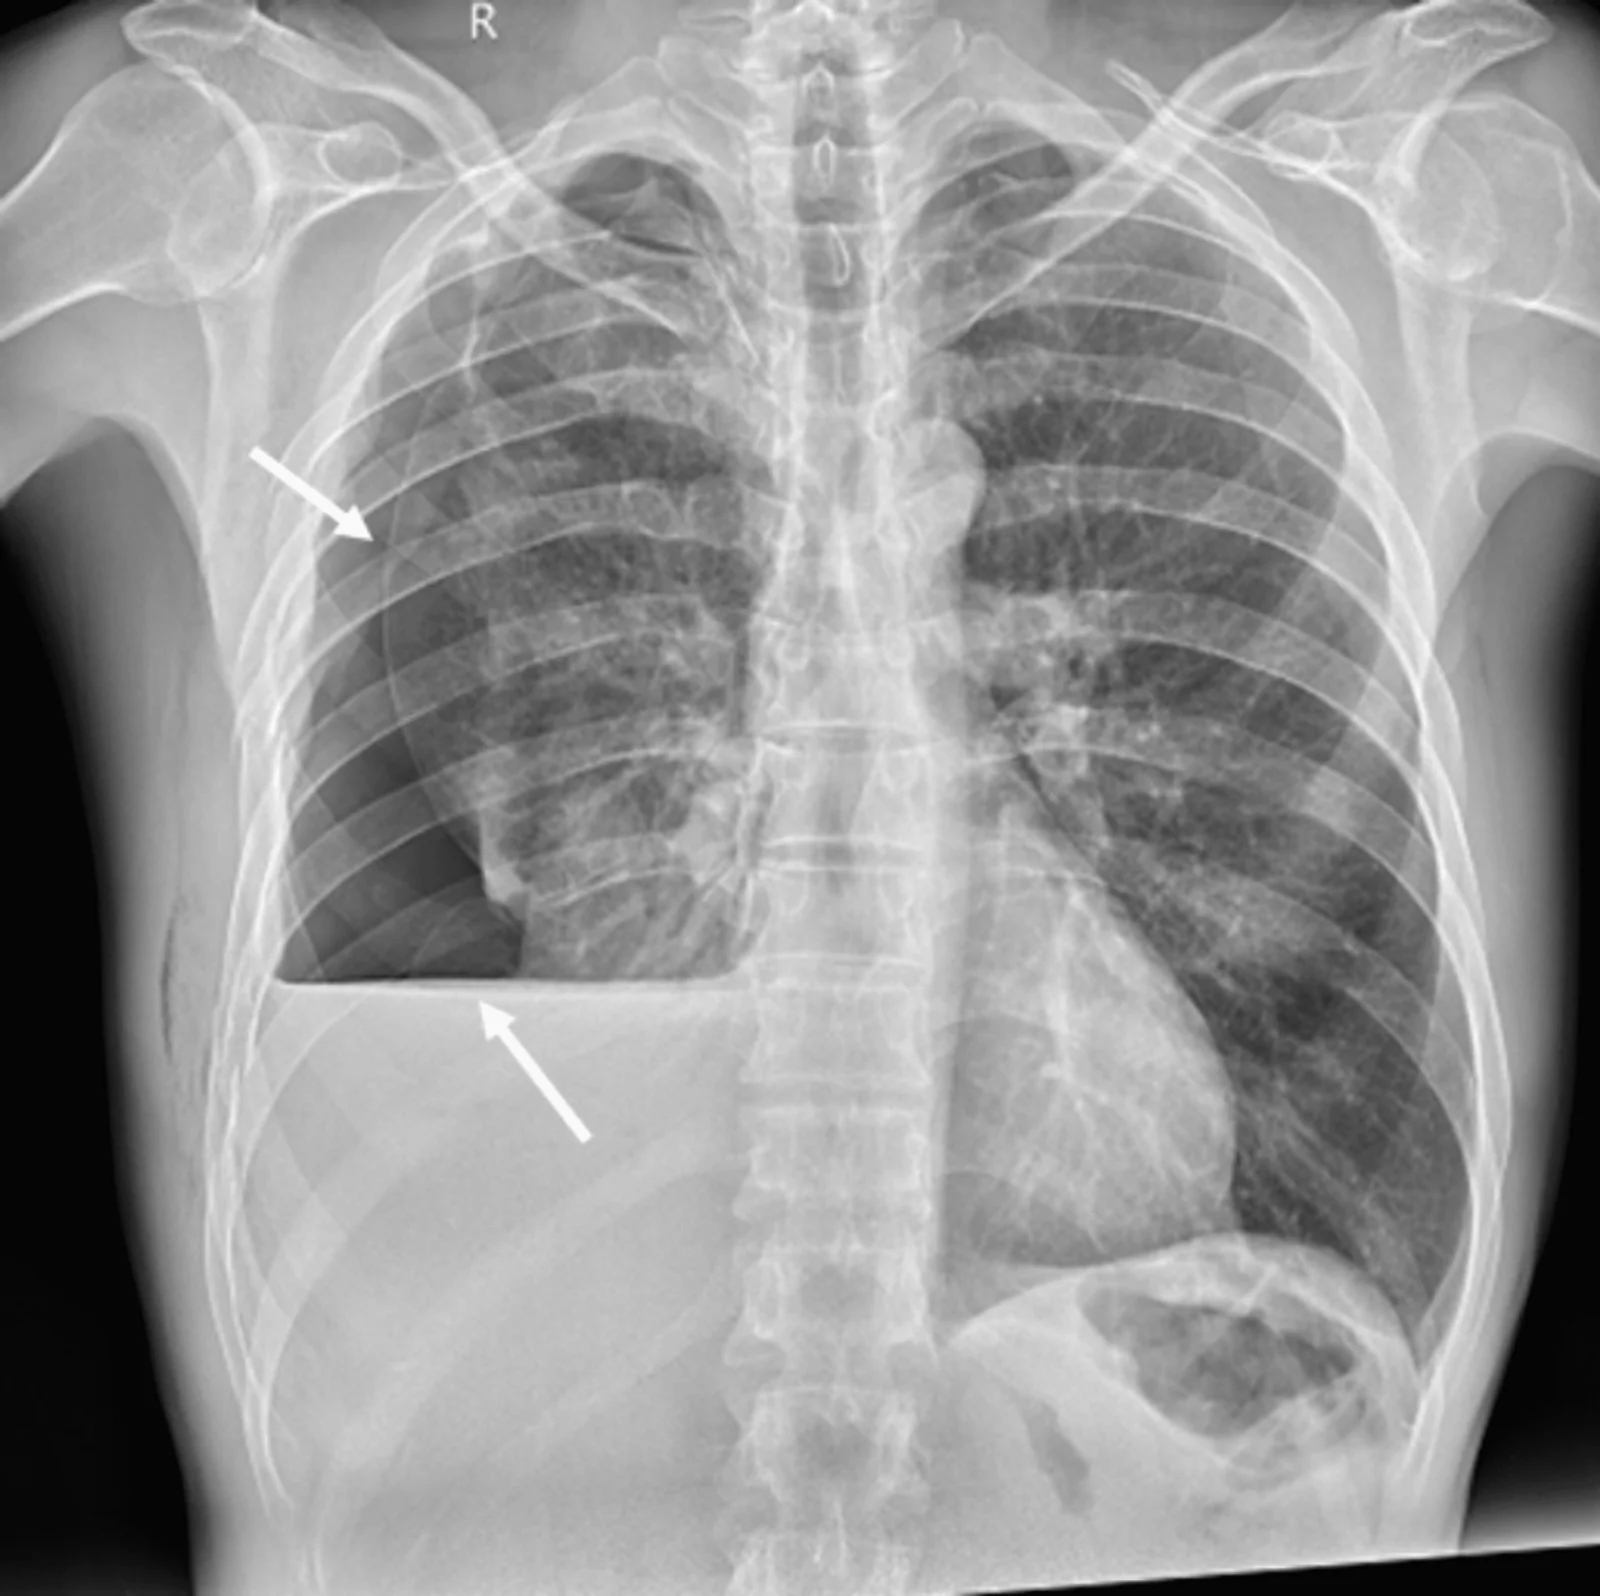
Upright anteroposterior chest radiograph obtained on day one of admission. Compared to the first radiograph, this chest radiograph demonstrates a right-sided pneumothorax with associated pleural fluid. The upper arrow indicates the visceral pleural edge of the collapsed right lung, while the lower arrow highlights the sharp, horizontal air-fluid level of the hydrothorax. The cardiac border is displaced to the left side, indicating a shift in the mediastinum.
